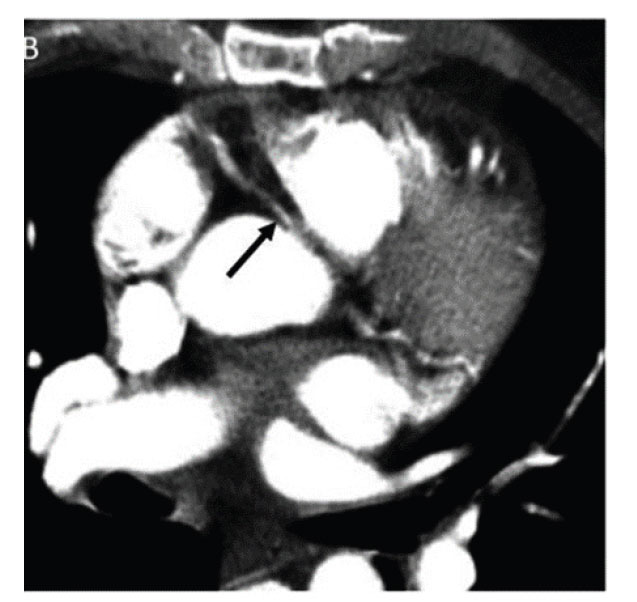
Axial contrasted CT scan of the chest showing an anomalous RCA arising from the left sinus of Valsalva coursing between the aorta and pulmonary artery with the proximal RCA with an oval/slit-like appearance suggesting an intramural course. Reproduced with kind permission from Dr Clay: Clay KJ, Hamid A, Deere BP. Two Cases of Highly Symptomatic Interarterial Anomalous Right Coronary Arteries. Cureus. 2023; 15: e42761.
